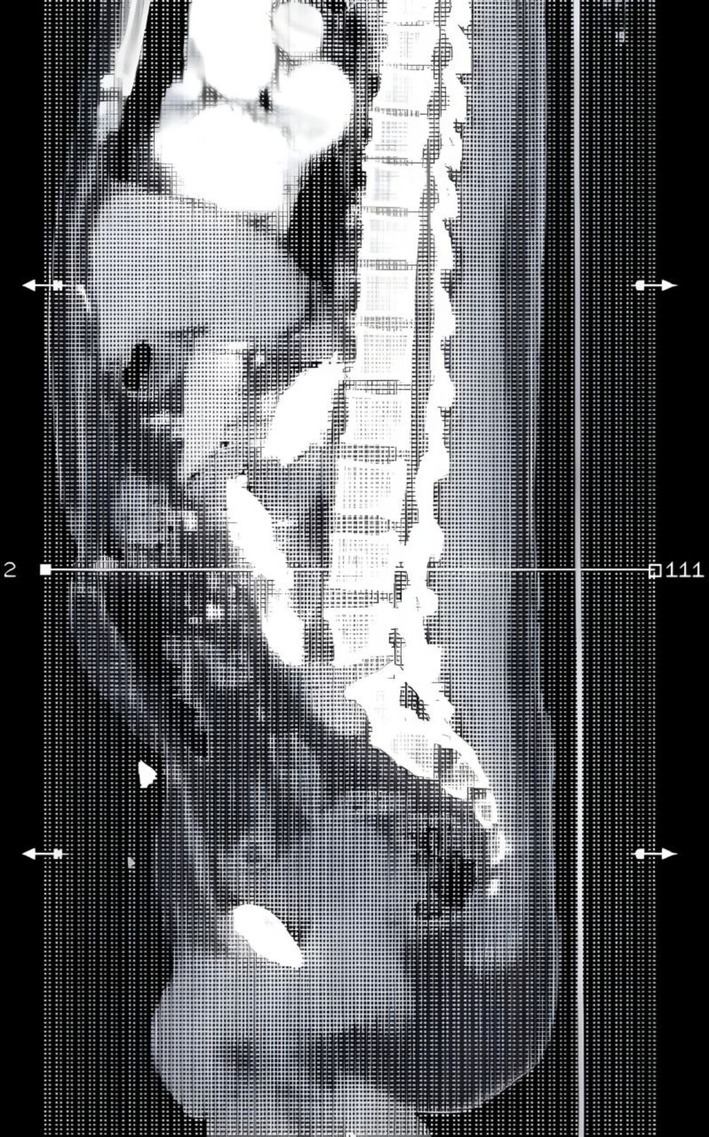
Sagittal reconstruction from a contrast‐enhanced computed tomography angiography (CTA) of the abdomen demonstrating a large infrarenal abdominal aortic aneurysm (AAA) with signs of rupture, evidenced by the disruption of the posterior aortic wall and retroperitoneal hematoma. The aorta is in close contact with adjacent bowel loops, raising suspicion for a secondary aorto‐enteric fistula. Loss of the fat plane between the aorta and bowel and possible air or contrast adjacent to the aneurysm suggest fistulous communication.
